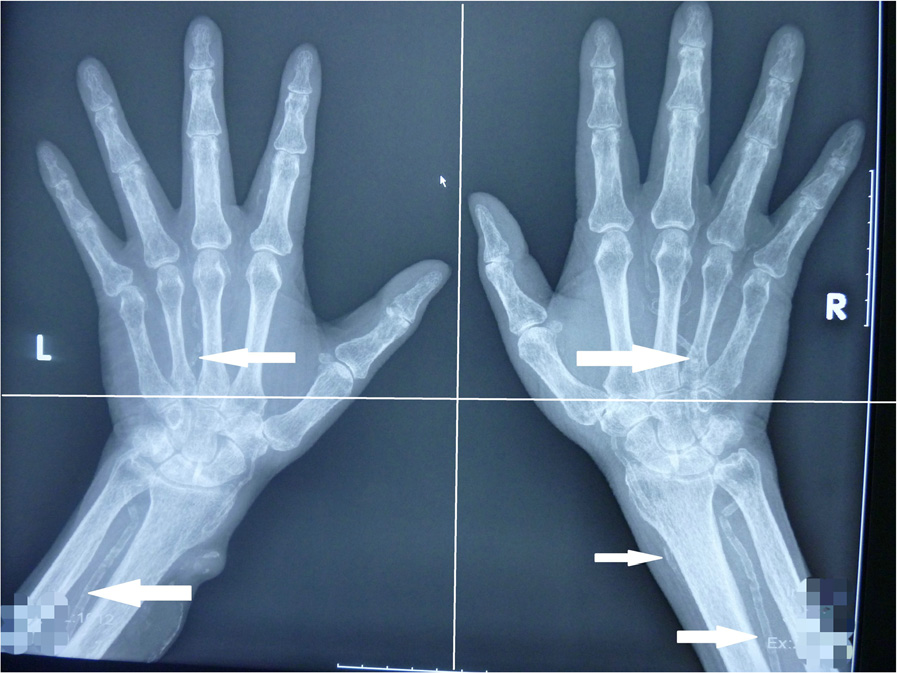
Vascular calcification in radiographs of the hand.
